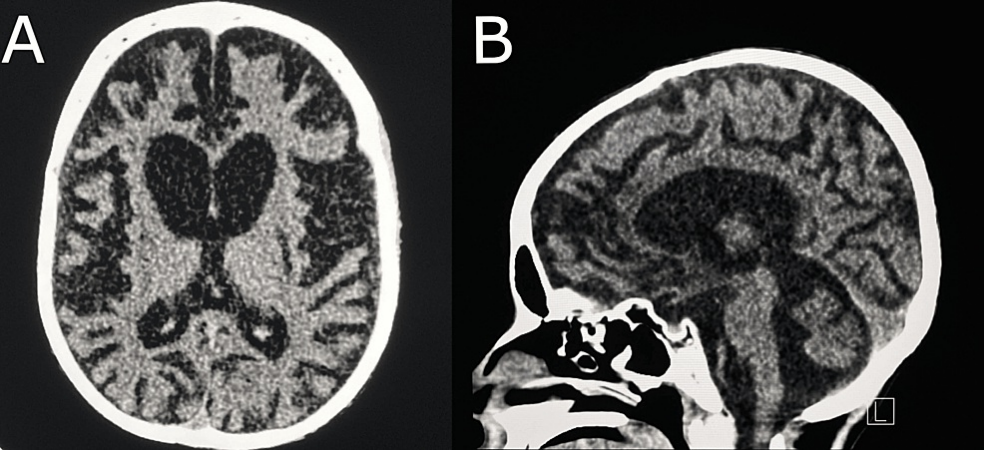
Axial plain brain CT image showing bilateral diffuse brain parenchymal volume loss, disproportionate to the patient's age (A), which resulted in dilatation of the ventricles and cortical sulci (B).
